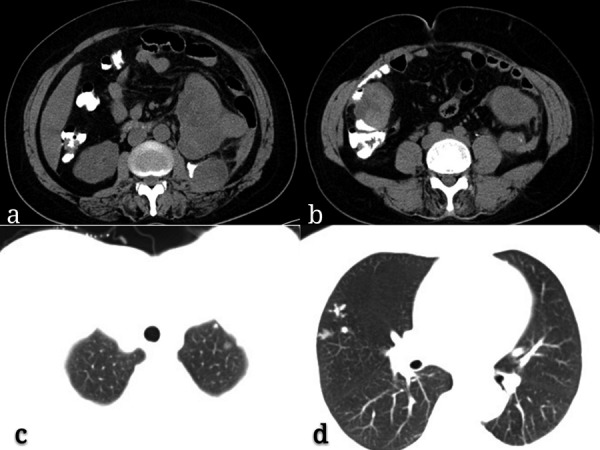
a, b: Unenhanced computed tomography images showed recrudescence and abdomen metastases. c, d: Multiple lung metastases are shown.
